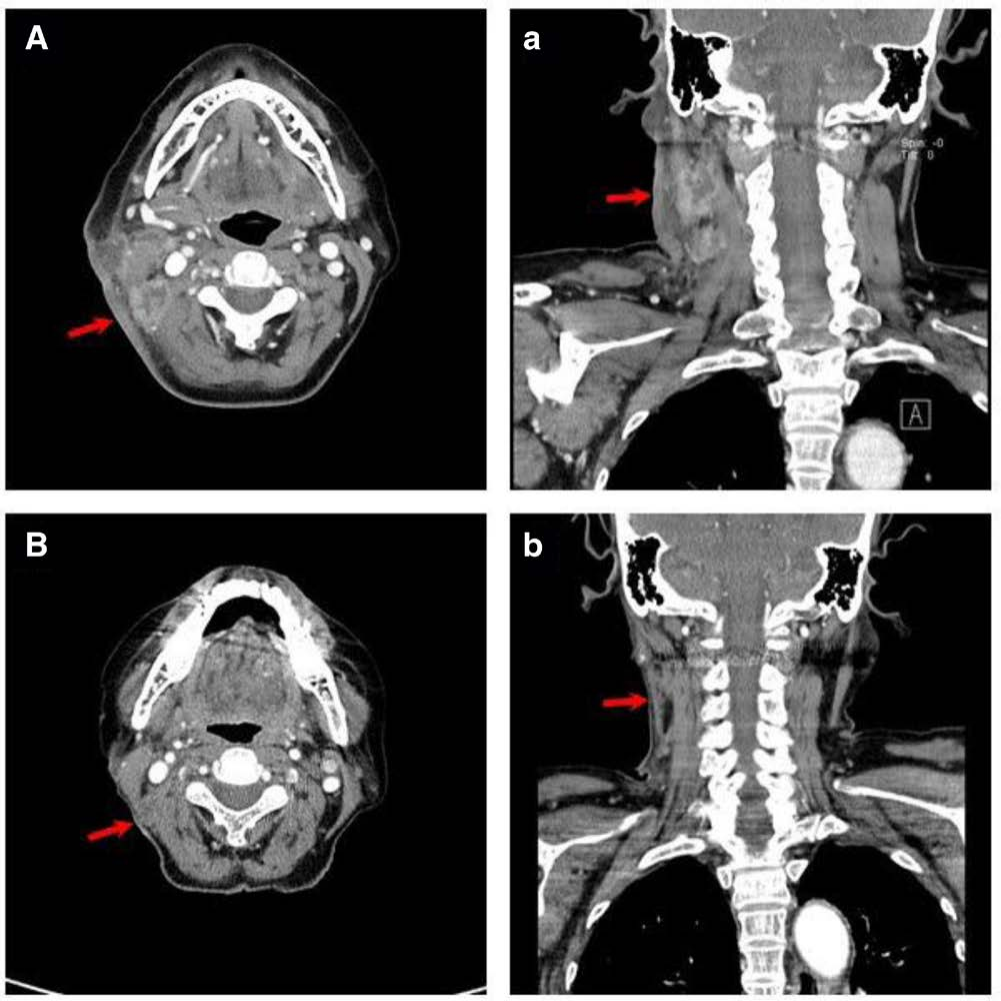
Computed tomography images of neck comparing the cervical lymph nodes before and 2 mo after starting TB medication. (A) A mass with a necrotic center around the right SCM muscle. (B) Posttreatment CT neck image, showing that the necrotic lymph nodes have reduced in size and significantly improved. CT = computed tomography, SCM = sternocleidomastoid, TB = tuberculosis.
